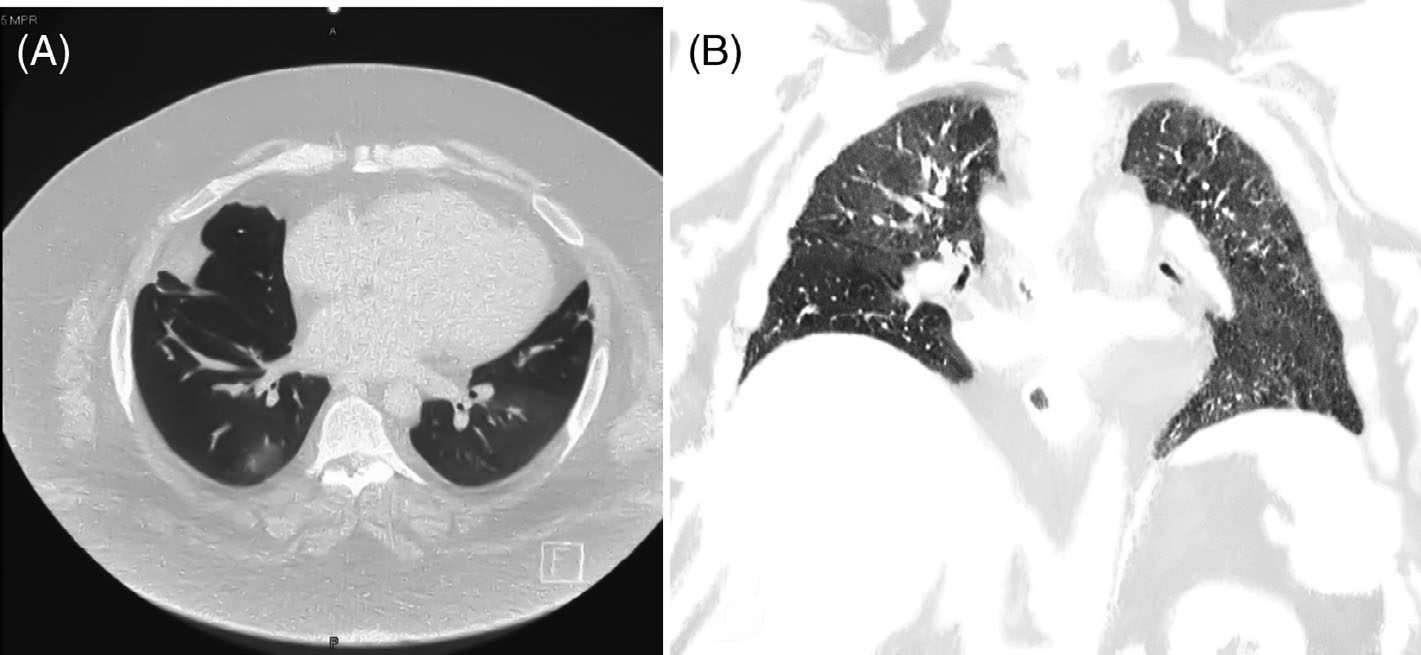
One‐year post‐initial improved computed tomography thorax, although there is still evidence of ongoing alveolar haemorrhage with bilateral ground‐glass changes. (A) Axial view. (B) Coronal view
